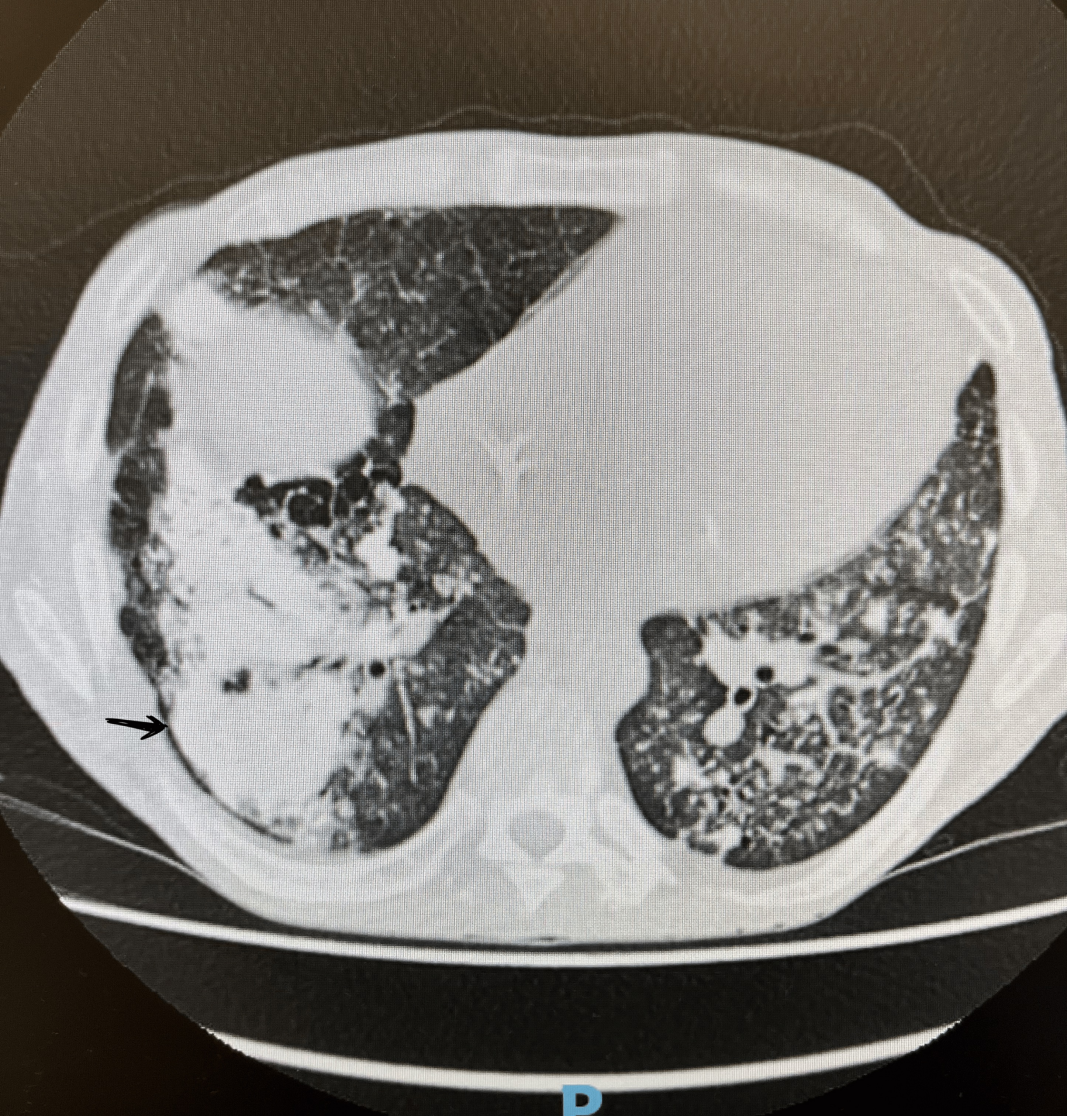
Chest CT demonstrating widespread bilateral ground-glass opacities with interlobular septal thickening This finding, in the context of end-stage renal failure and severe mineral imbalance, is consistent with metastatic pulmonary calcification.
